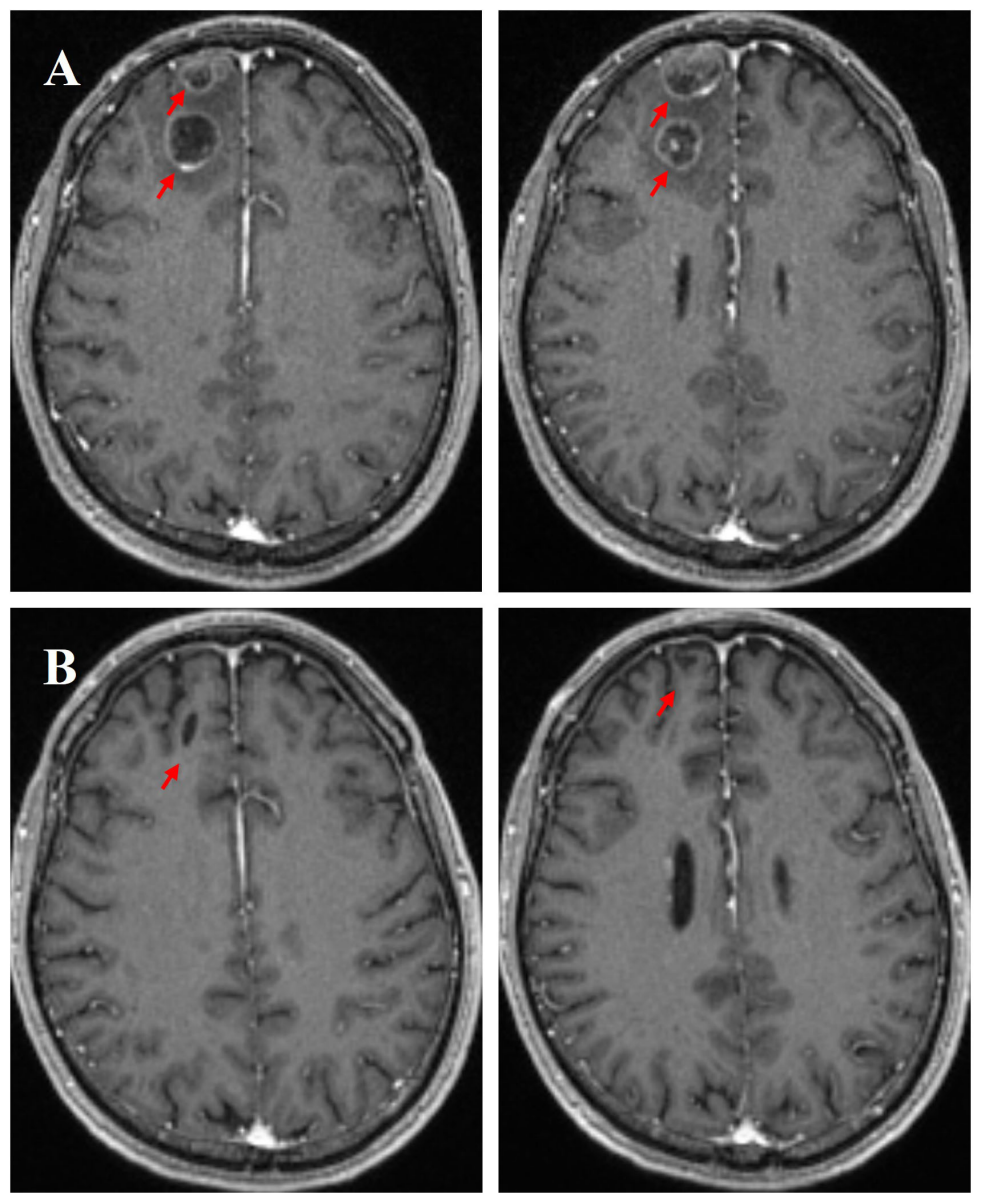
Enhanced magnetic resonance imaging (MRI) scans of the brain on October 28, 2023, further confirmed new brain metastases (A). Following palliative radiotherapy, an MRI evaluation of the brain metastatic lesions was performed on February 23, 2024, showing a complete response (CR) (B).
